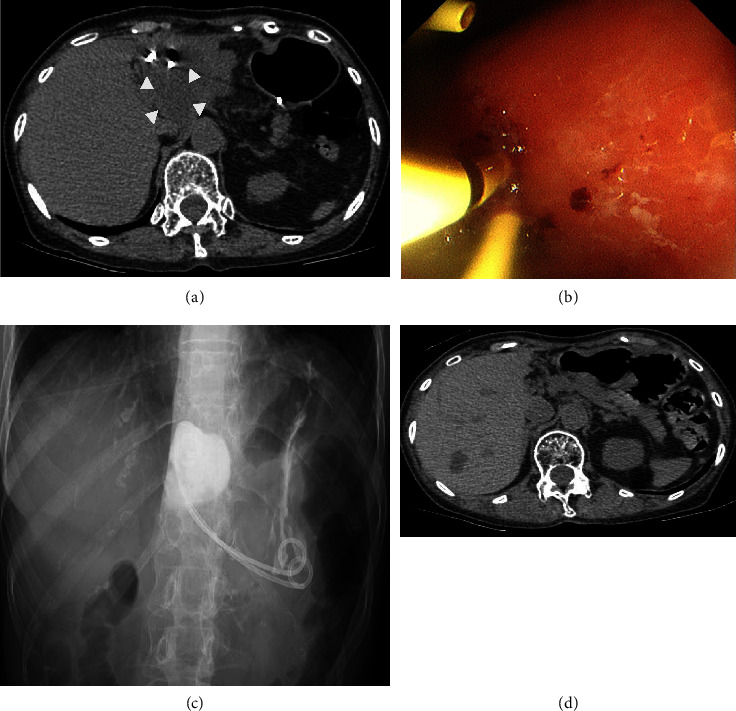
Computed tomography (CT) scan after the first sclerotherapy showing the shrunken liver cyst (a) (arrowheads). We exchanged the external tube for an internal stent (two internal stent insertions) ((b) endoscopy; (c) X-ray). A CT scan showed no recurrence of the liver cyst for 6 months after stent removal (d).
